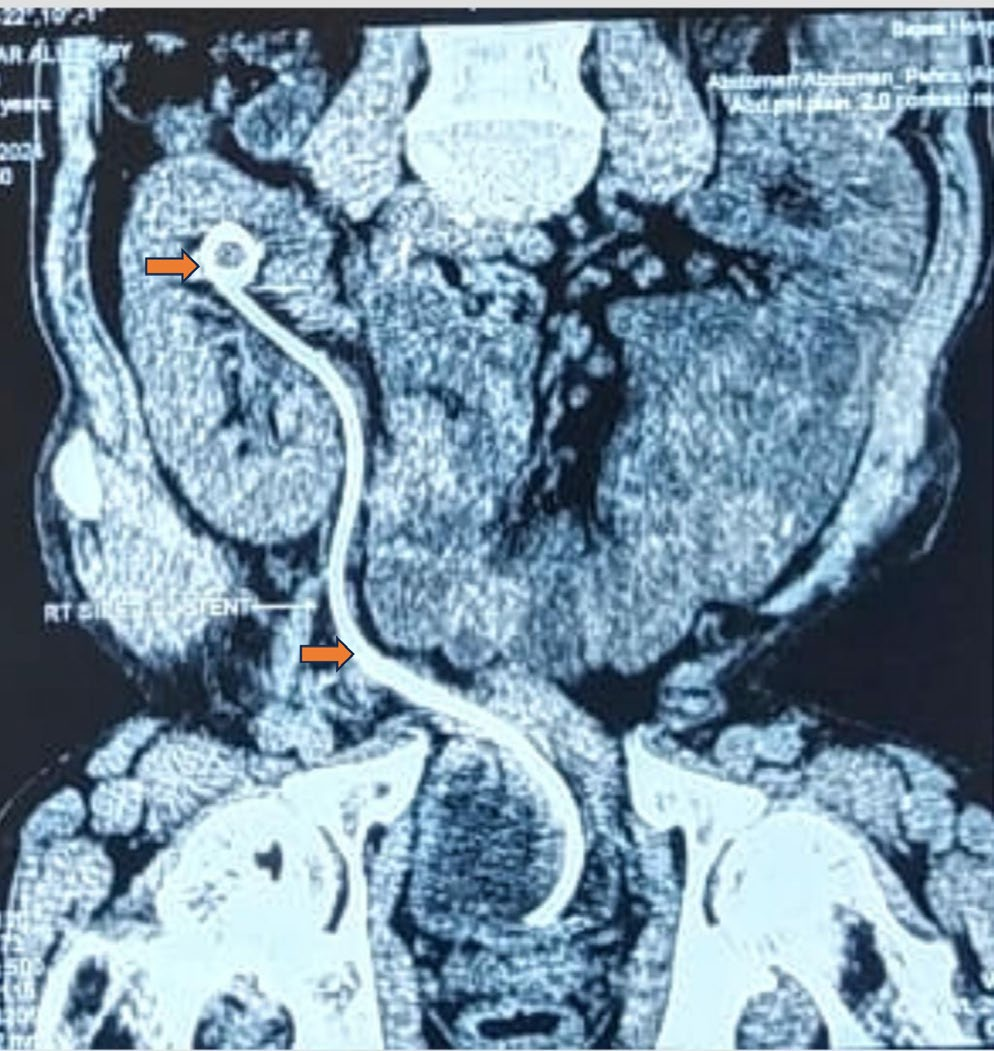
CT scan revealed encrustation at the upper end of the DJ stent.
